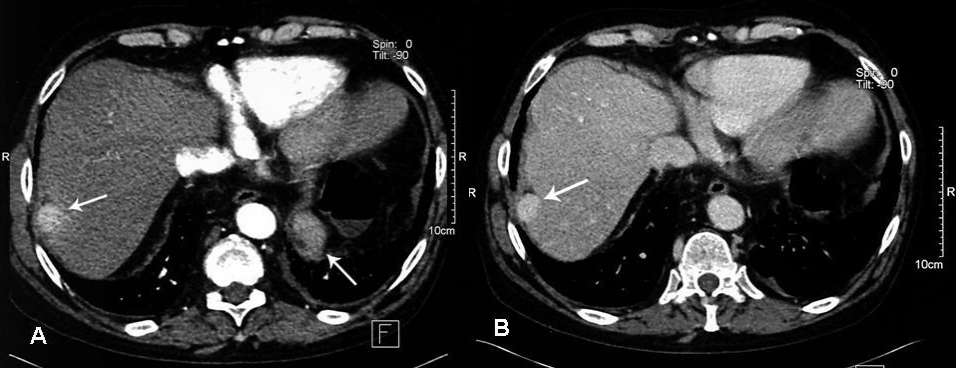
CT scan. (A) Axial IV contrast enhanced CT. Arterial phase image showing a 2 × 2.7 cm hypervascular, subcapsular nodule in segment VII of the liver. (B) Portal venous phase image.
